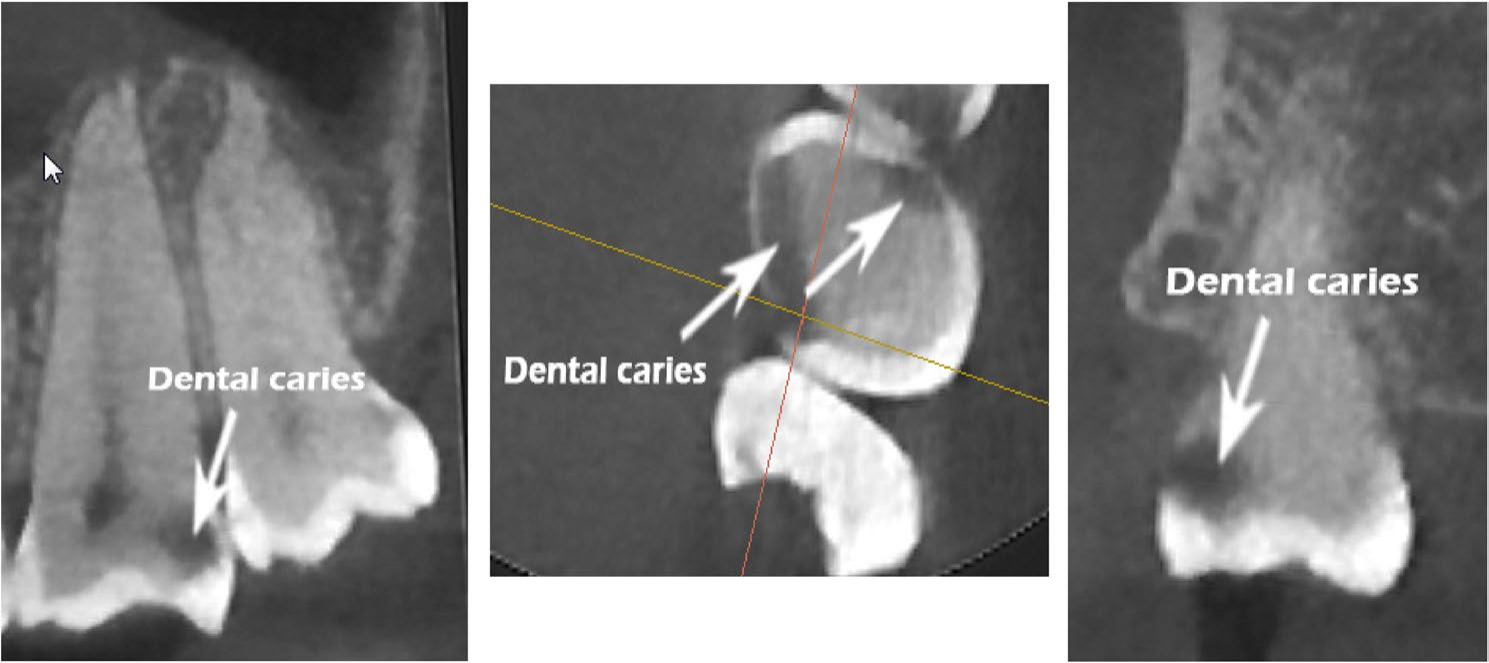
CBCT images showing dental caries of M2 in sagittal, axial, and coronal views
